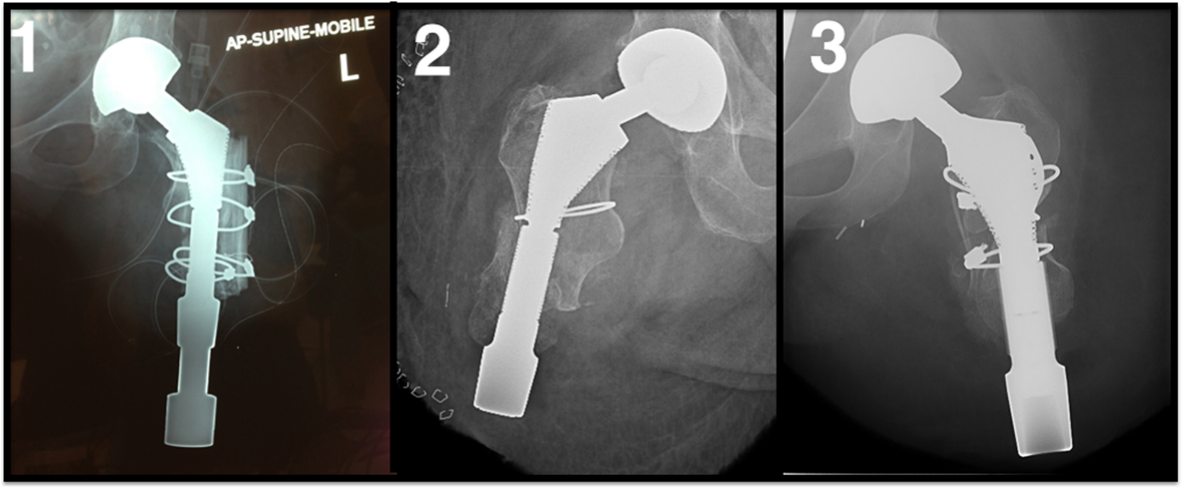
Radiographs at follow-up for case 1, 2 and 3
